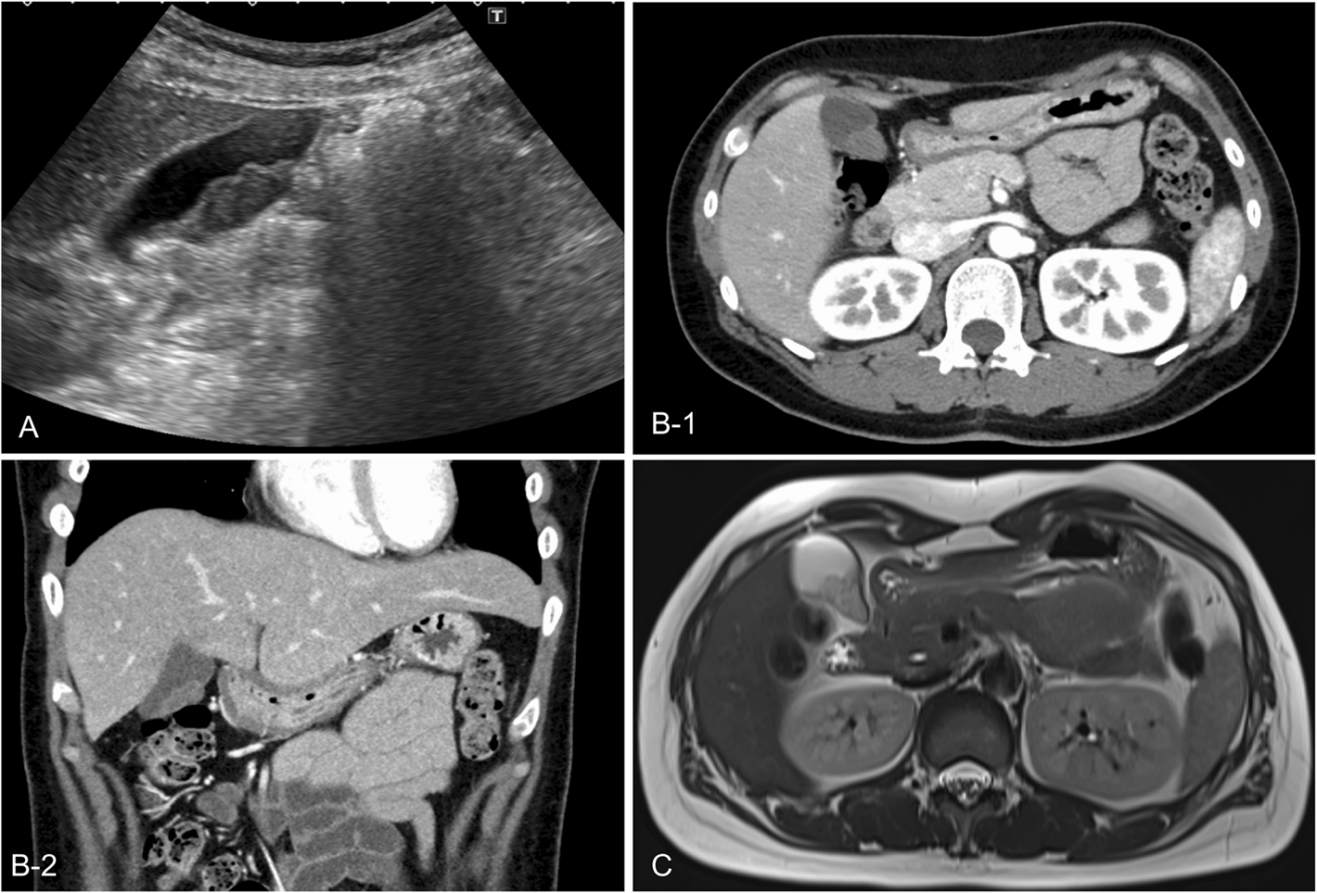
a US image showing a mass in the gallbladder. b-1 CECT scan image showing a mass in the gallbladder wall that takes up contrast. b-2 CECT scan image showing a mass in the gallbladder wall that takes up contrast. c MRI T2 image showing a gallbladder lesion with hypointensity
